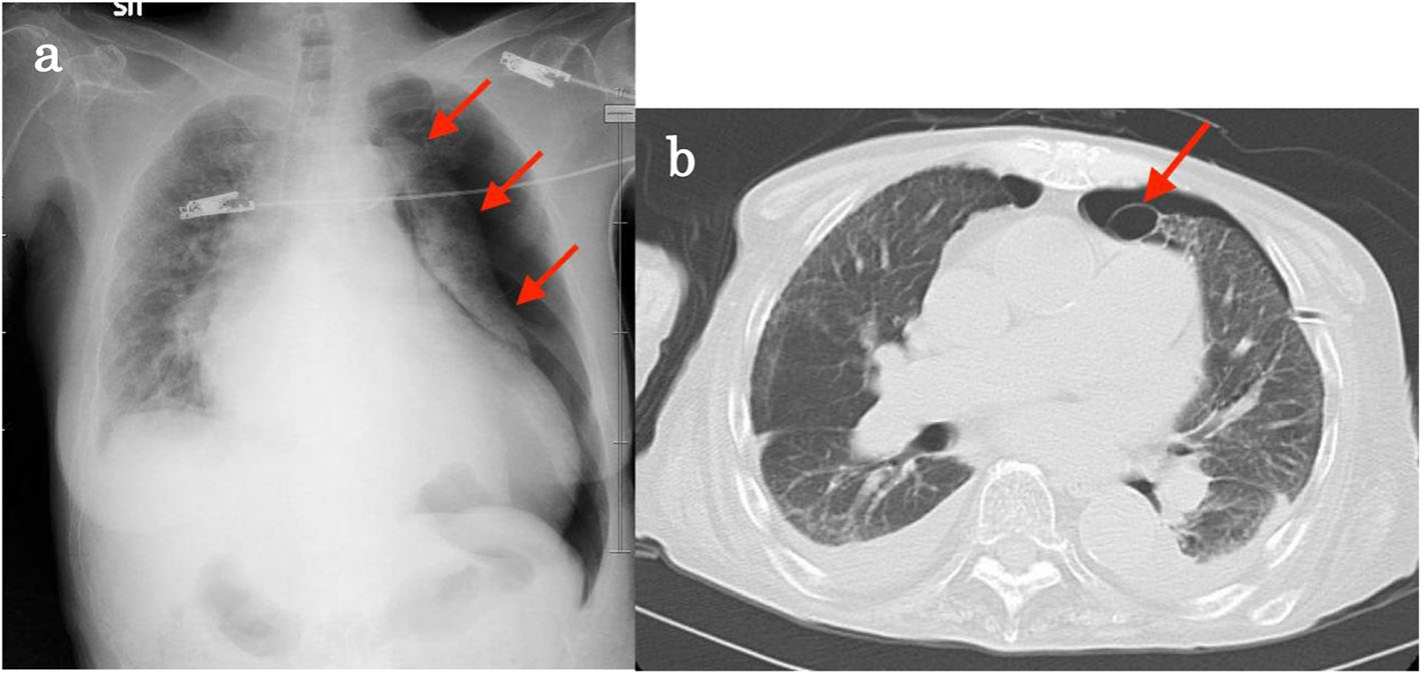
Preoperative images of the patient. a Chest X-ray shows the collapsed lung (red arrows). The left pneumothorax is severe. b Computed tomography shows a bulla of the left upper lobe behind the sternum (red arrow). The bulla was suspected as the cause of the pneumothorax.
